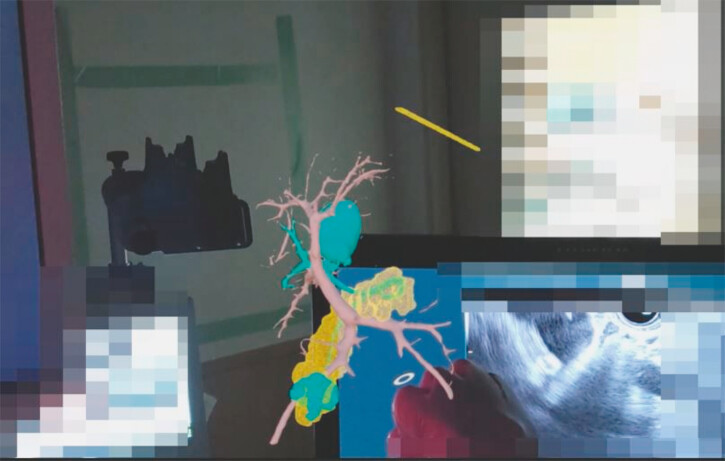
Simultaneous display of the endoscopic ultrasonography image and a 3D hologram through the see-through goggles.
